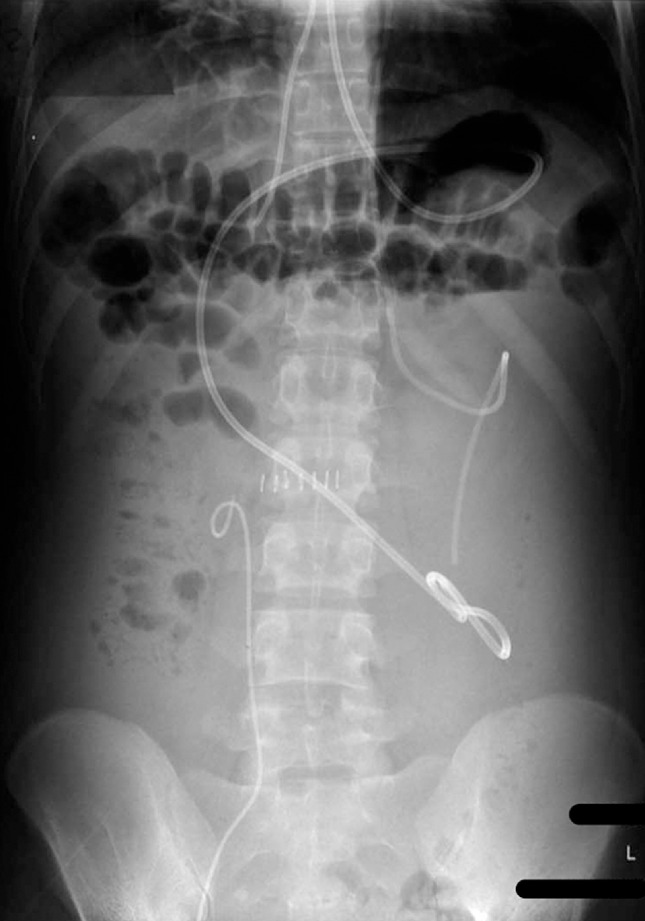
Post-procedure X-ray of the abdomen showing a nasocystic drain, right ventriculo-pleural shunt, left ventriculoperitoneal shunt, and right double J stent.
